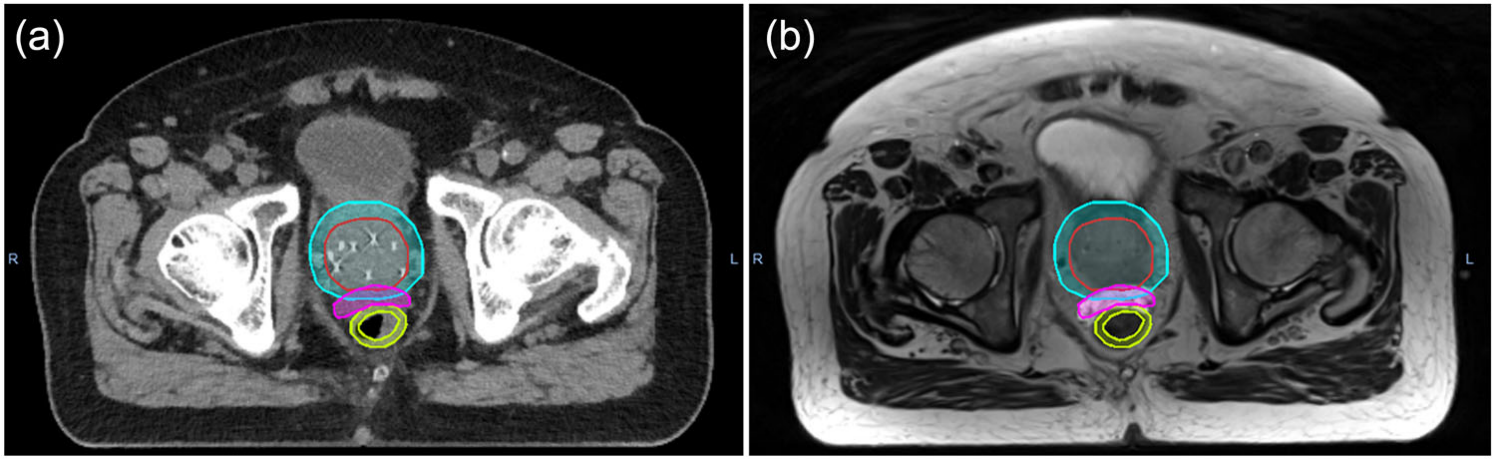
An example of computed tomography (CT) (a) and magnetic resonance imaging (MRI) (b). The hydrogel spacer (pink) was inserted between prostate (red) and rectal wall (light green). PTV_45_ (green) was prostate (red) with expansion of the margins.
